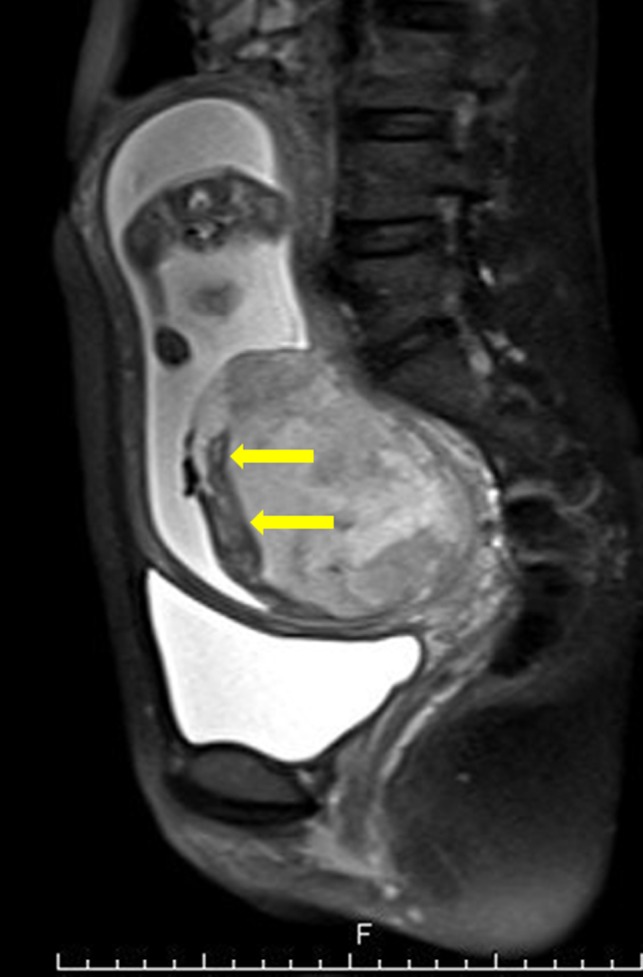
MRI T2‐weighted images at gestation of 24 wk and 3 d. MRI shows that the placenta is heterogeneous and enlarged. Arrows indicate fluid‐fluid level in the image. Placenta accreta was not observed
